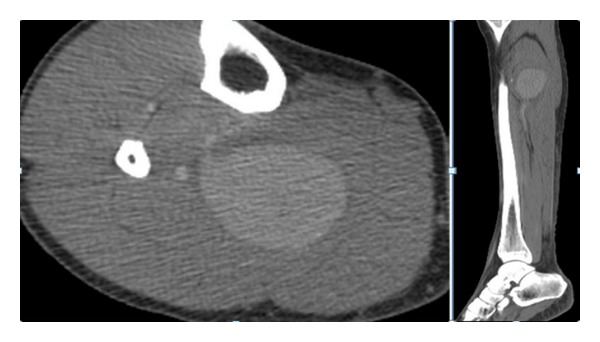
The giant posterior tibial artery aneurysm was diagnosed by angio-CT scan.
